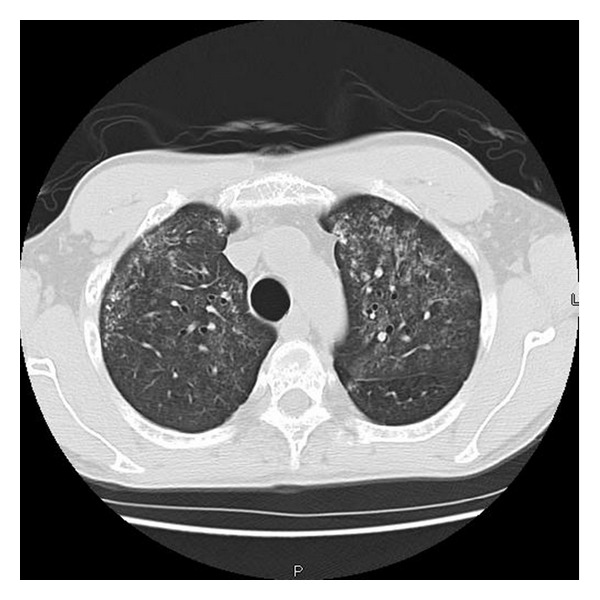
Chest CT showing ground-glass nodular opacities, with poorly defined nodules.
